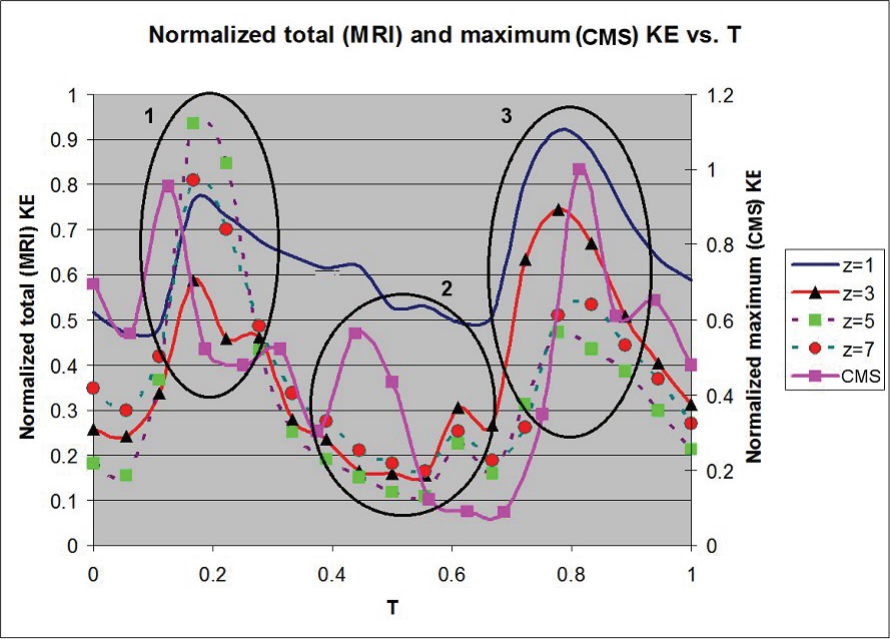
The comparison of Kinetic Energy (KE) between the 4-D PC-MRI system and CMS. Normalized total KE at different slices and maximum KE at slice z = 0.56, obtained from the 4-D PC-MRI system and CMS, respectively.
